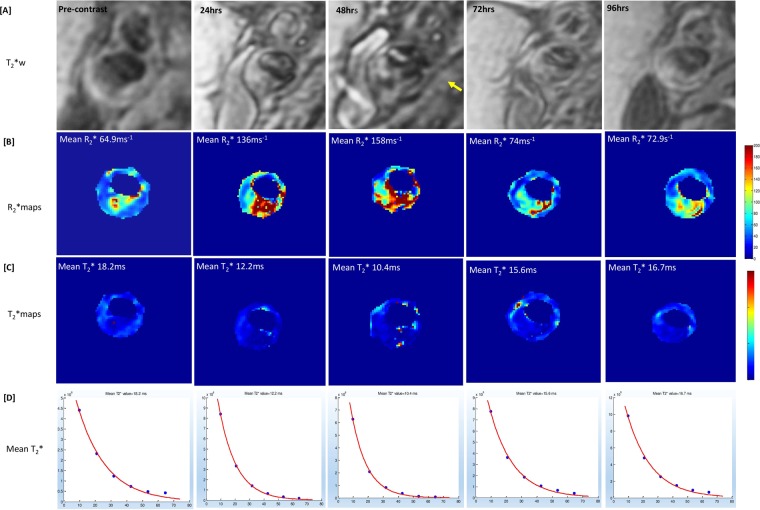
Ferumoxytol-enhanced magnetic resonance images of carotid atheroma with corresponding T2*/R2* maps and T2* graphs at baseline and 24, 48, 72 and 96 hrs of ferumoxytol administration demonstrating the maximum R2* increase of the atherosclerotic plaque after ferumoxytol administration at 48 hrs. [A] Images of the multi-echo T2*/R2* MR sequence at all 5 time points with signal void in carotid atheroma (yellow arrow) visible at 48 hrs following ferumoxytol administration. [B] Shows corresponding R2* maps (mean R* values) pre-contrast and at 24 hr, 48 hr, 72 hr and 96 hr following ferumoxytol administration. Note the maximum R2* increase of the atherosclerotic plaque after ferumoxytol administration at 48hrs, reflecting USPIO uptake. [C,D] Shows the corresponding T2* maps and graphs demonstrating maximum decrease in T2* values at 48 hr.
